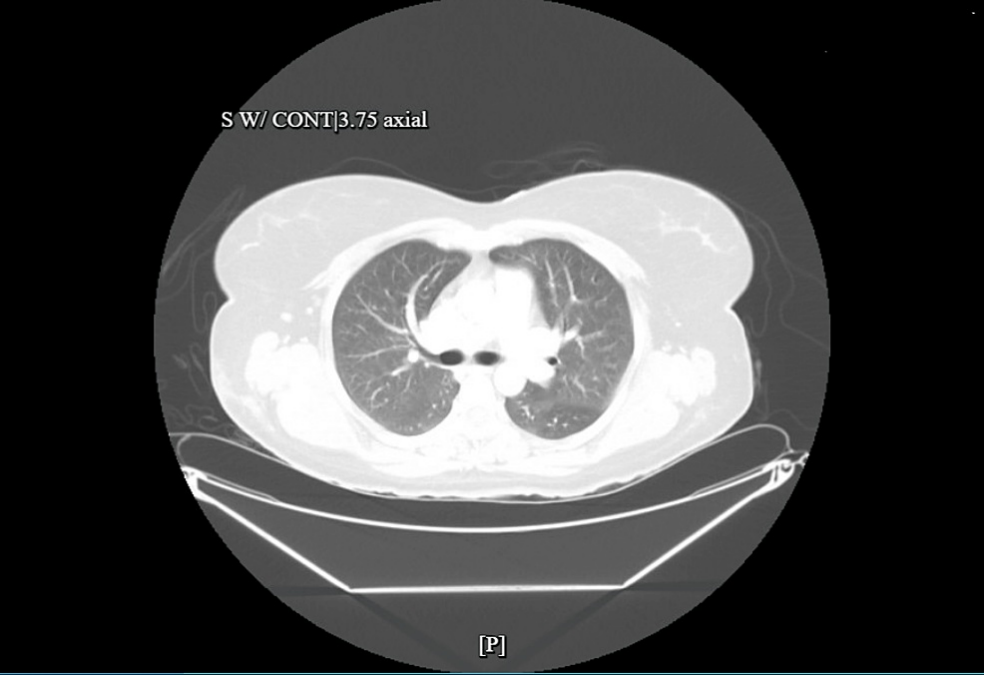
CT scan performed one week after admission demonstrating resolution of opacities previously seen in bilateral lung zones
